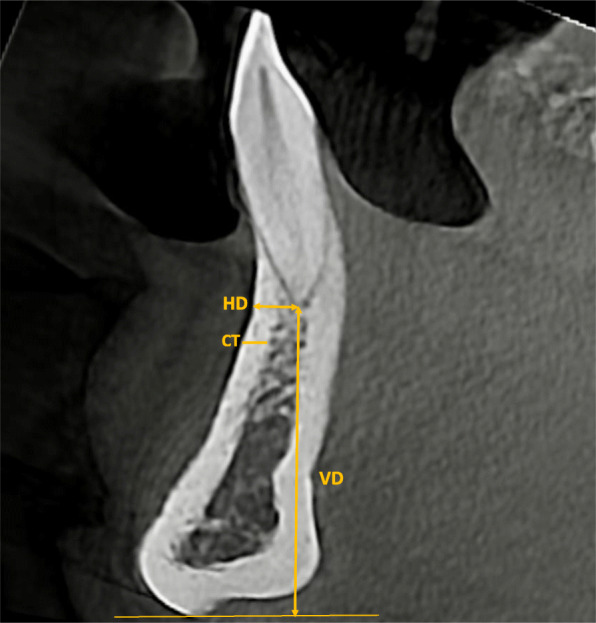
Measurement of horizontal (HD) and vertical (VD) bone dimensions and cortical bone thickness (CT) of the mandibular symphysis on a multiplanar section of the mandibular central incisor region
